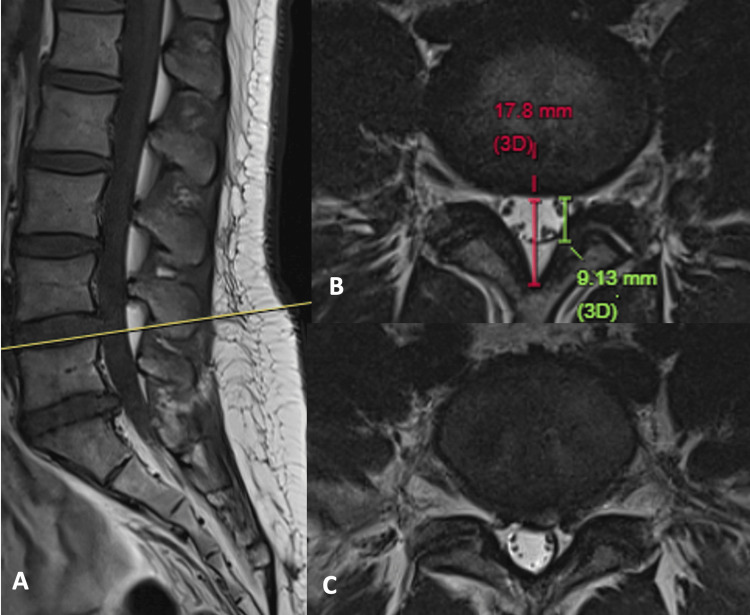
MRI of the lumbosacral spine of a 25-year-old woman with buttock pain radiating to the left leg. (A) Sagittal T1-weighted image shows prominent epidural fat, especially from L2 to S2 levels. (B) Axial T2-weighted image at L5 superior endplate shows a normal dural sac (dura/fat ratio = 1.05, fat% = 49%), mild SEL. (C) Axial T2-weighted image at the L5/S1 level shows posterior disc protrusion, causing bilateral lateral recess stenosis touching descending S1 nerve roots. This may explain patient’s radiculopathy. MRI: magnetic resonance imaging; SEL: spinal epidural lipomatosis.
